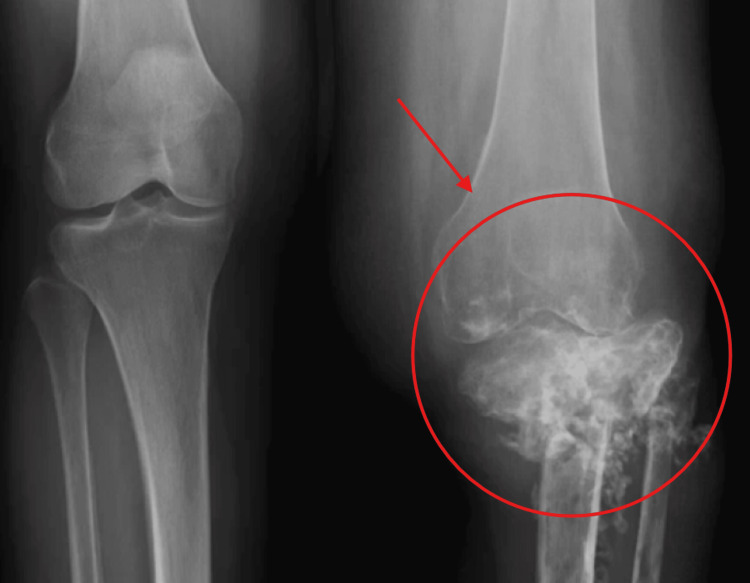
Plain radiograph of the bilateral knee and tibia/fibula (current)
